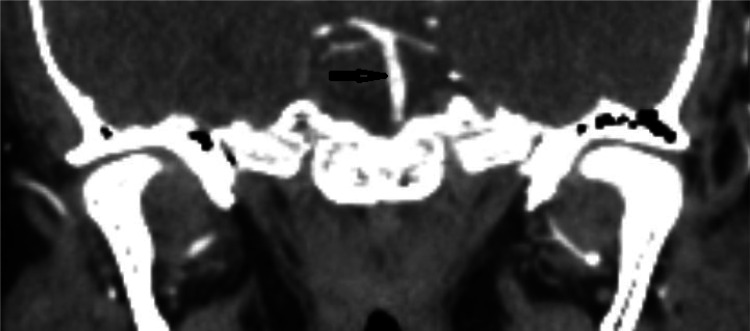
CTA coronal section taken at 3-months post-diagnosis.
